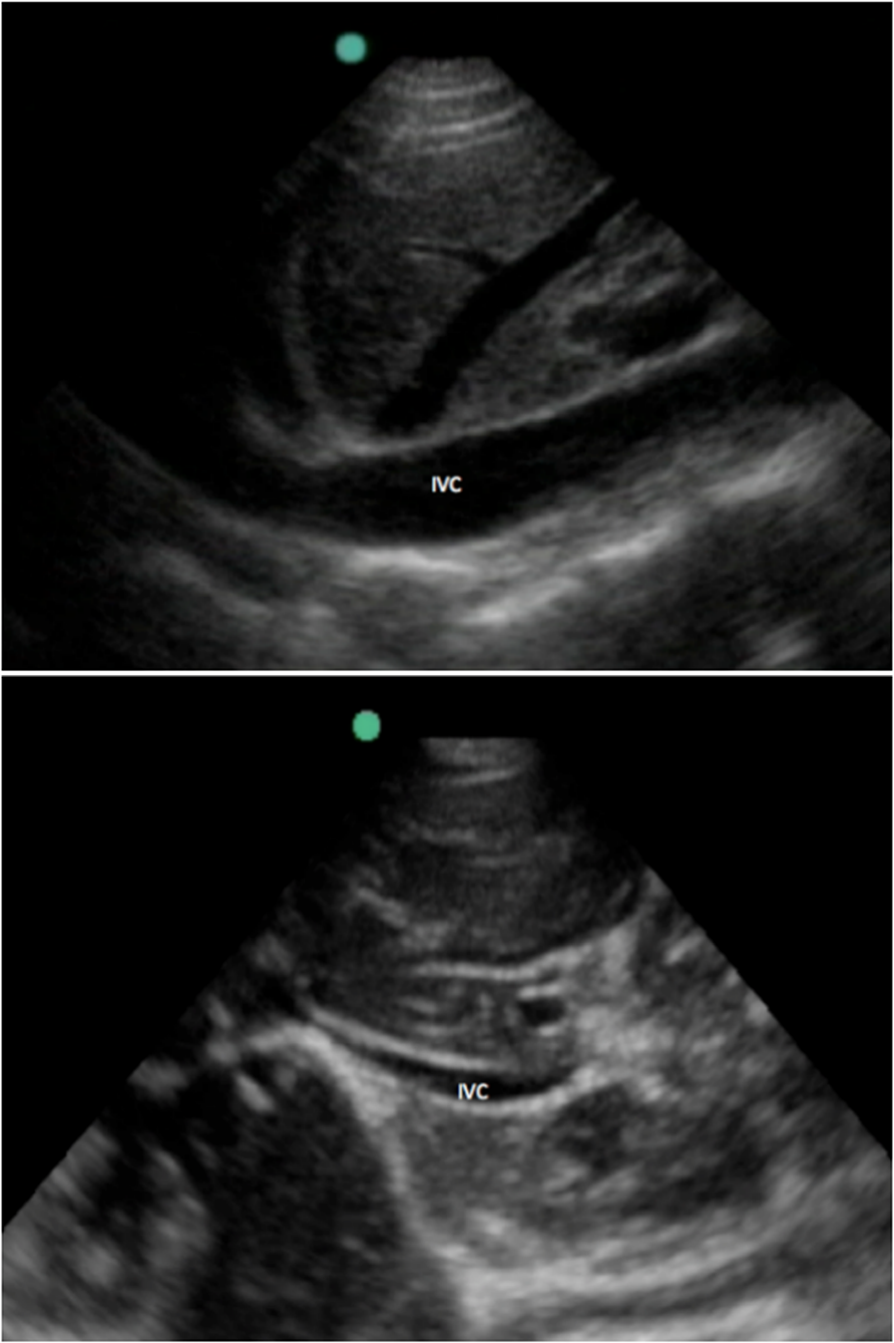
Ultrasound image of the longitudinal inferior vena cava collapsibility in expiration above and inspiration below. IVC, inferior vena cava.
